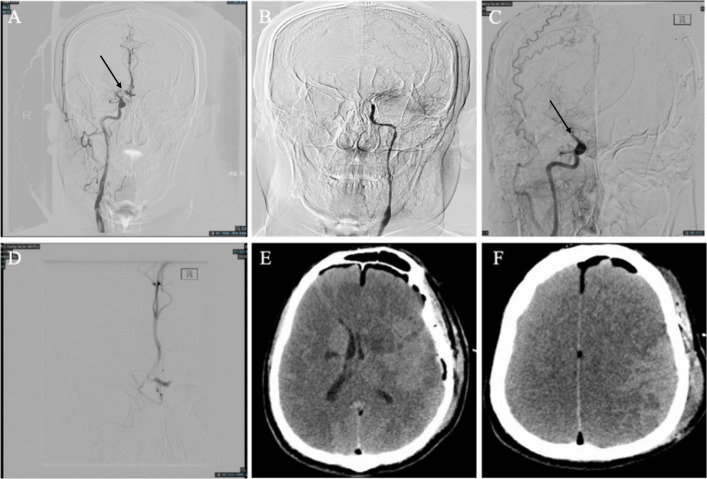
Major artery infarction with severe stenosis and occlusion. DSA and CT images of a 47-year-old male patient with MMD. A and (B) Right and left ICA angiographs; the arrow in A shows severe stenosis of the first part of the ACA (A1). C Right ICA angiograph after revascularization; the arrow shows occlusion of the ACA. D Microcatheter angiograph passing through A1 showing that acute occlusion occurred in A1. E, F CT scan 4 h after the operation showing a newly developed area of low density in the bilateral cerebrum, which is consistent with the A1 supply area
